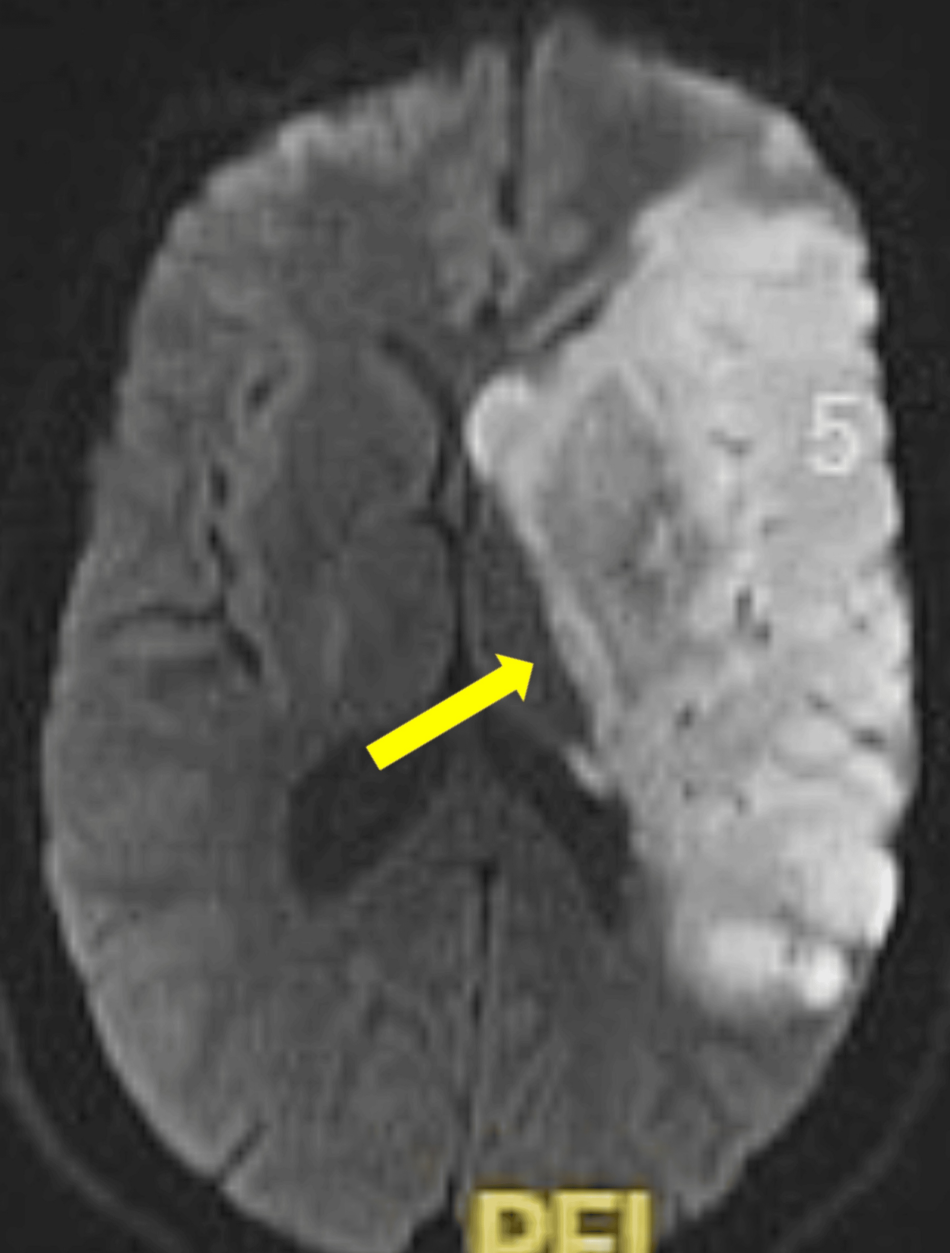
MRI brain showing the left MCA territory ischemic stroke MRI: magnetic resonance imaging, MCA: middle cerebral artery
